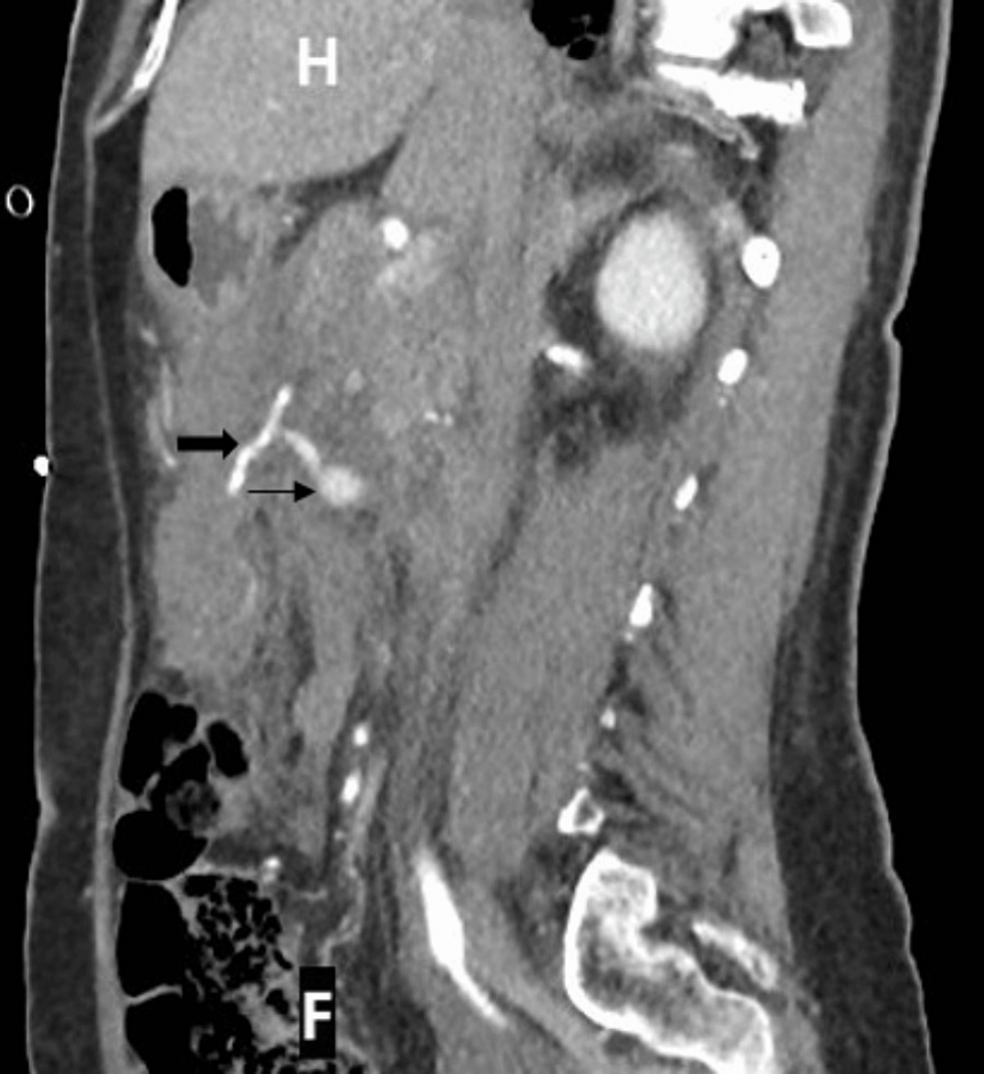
Arterial phase CT scan. Sagittal reformatted image demonstrating origin of pseudoaneurysm (thin arrow) from right colic branch of the superior mesenteric artery (thick arrow)
